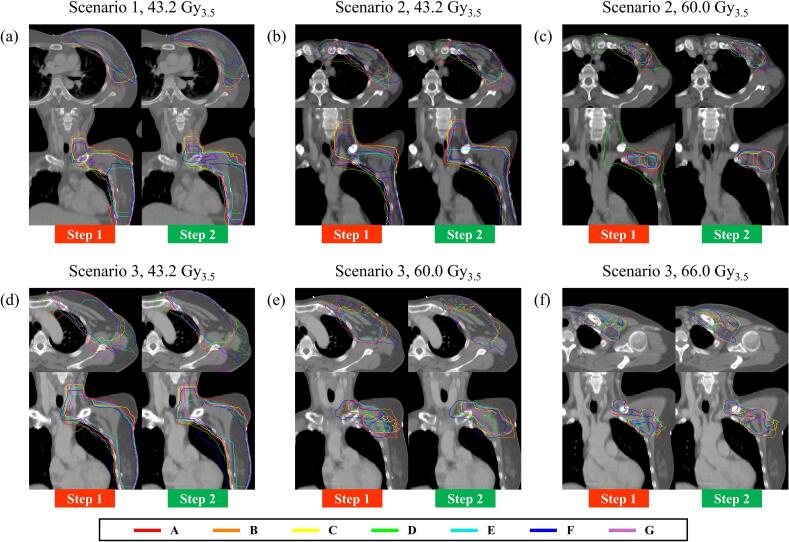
95% isodose lines for each participating institution by scenario and prescribed dose. Each set of CT images includes an axial view (top) and a coronal view (bottom), with comparisons between Step 1 (left) and Step 2 (right). Abbreviation: Gy_3.5_, equivalent dose in 2 Gy fractions (EQD2) with α/β = 3.5 Gy.
